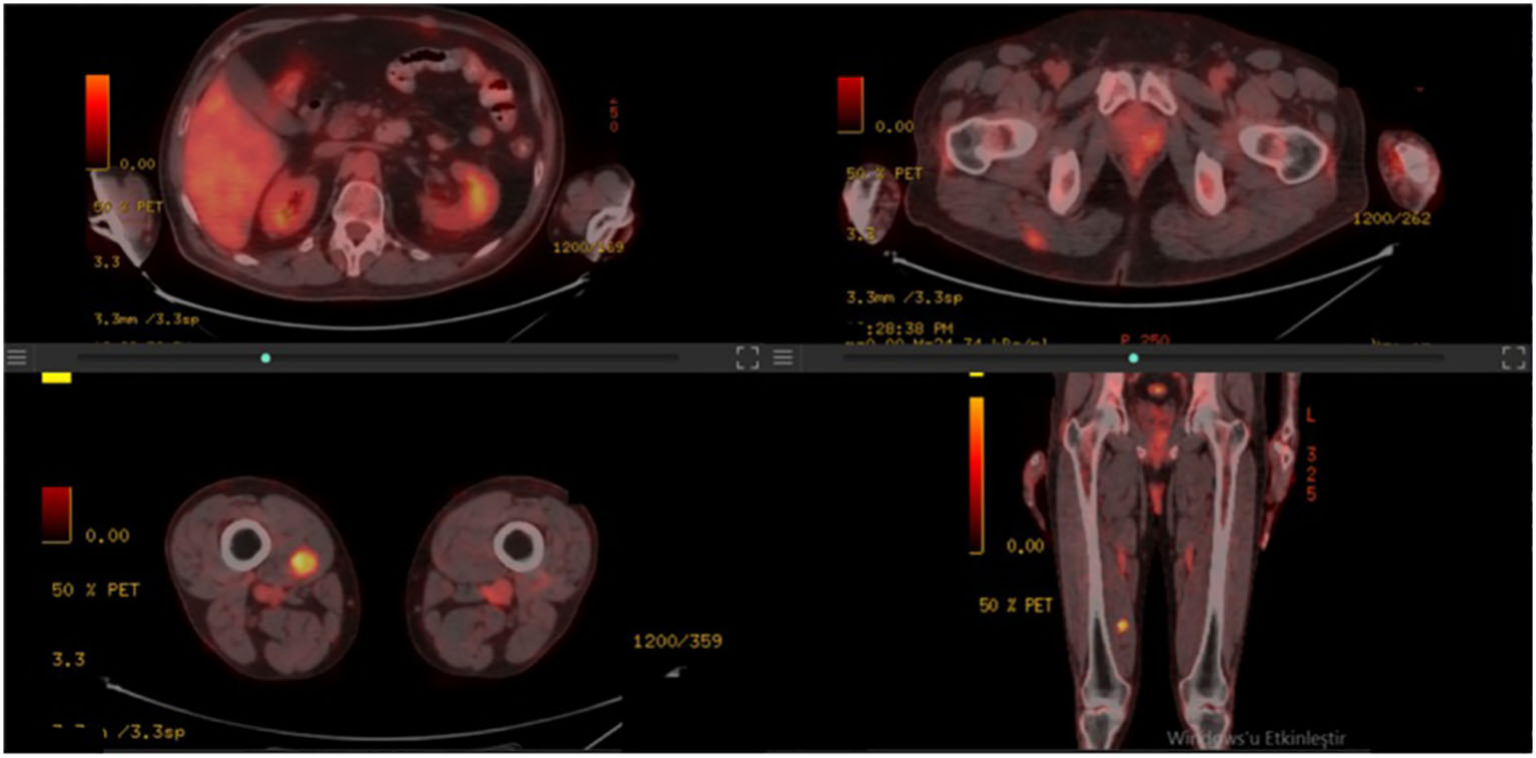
PET-CT images detecting metastatic disease.
